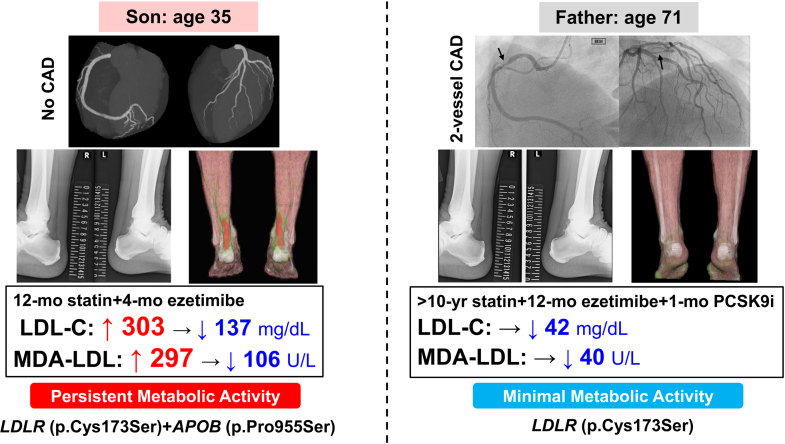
Differential Achilles Tendon Xanthoma Metabolic Activity: Impact of Genotype and Treatment Duration CAD = coronary artery disease; FDG-PET/CT = ^18^F-fluorodeoxyglucose positron emission tomography/computed tomography; LDL-C = low-density lipoprotein cholesterol; MDA = malondialdehyde-modified.
